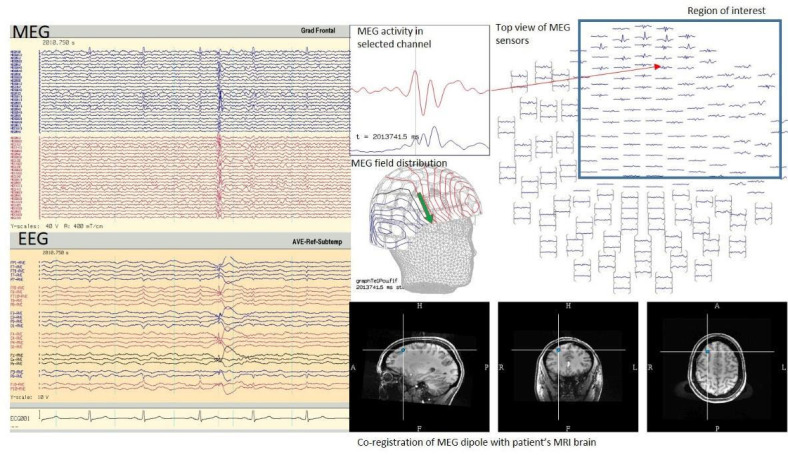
Tracings from magnetoencephalography at the top and from electroencephalography at the bottom and typical presentation of magnetoencephalography activity with magnetic field distribution and co-registration with the patient’s brain magnetic resonance image.
